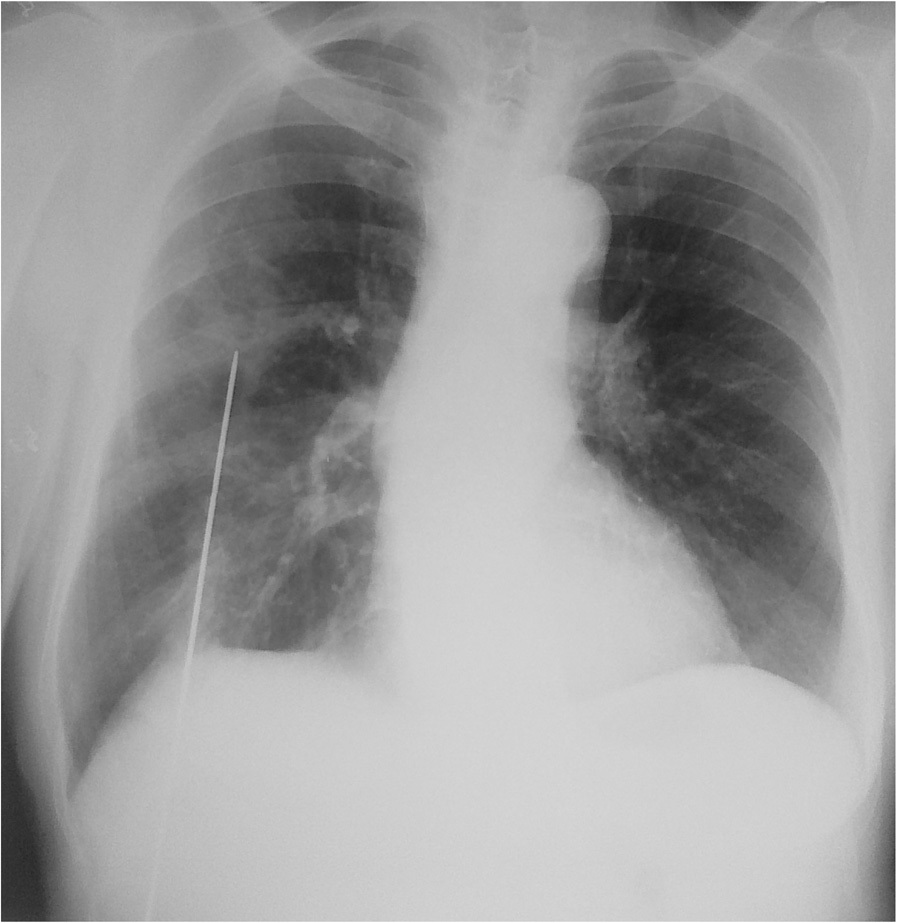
Initial chest X-ray showing a metallic foreign body extending from abdomen into chest
